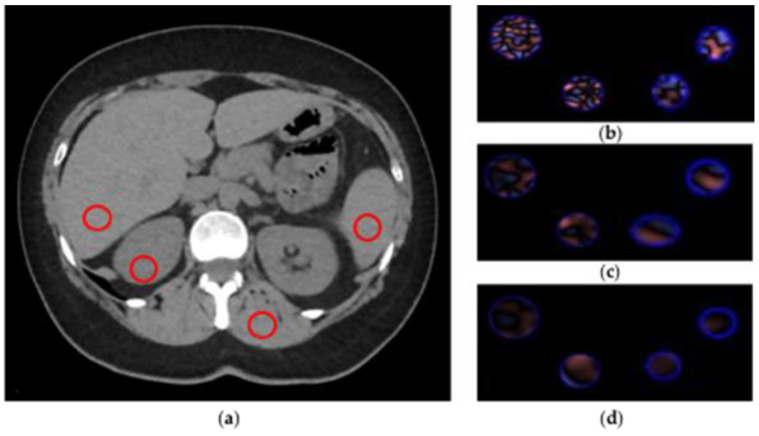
CT texture analysis (CTTA) process. (a) Regions of interest (ROIs) placement. (b–d) Filtration-histogram statistic-based method corresponding to fine, medium and coarse texture scale of ROIs (area 1 cm^2^), respectively.
